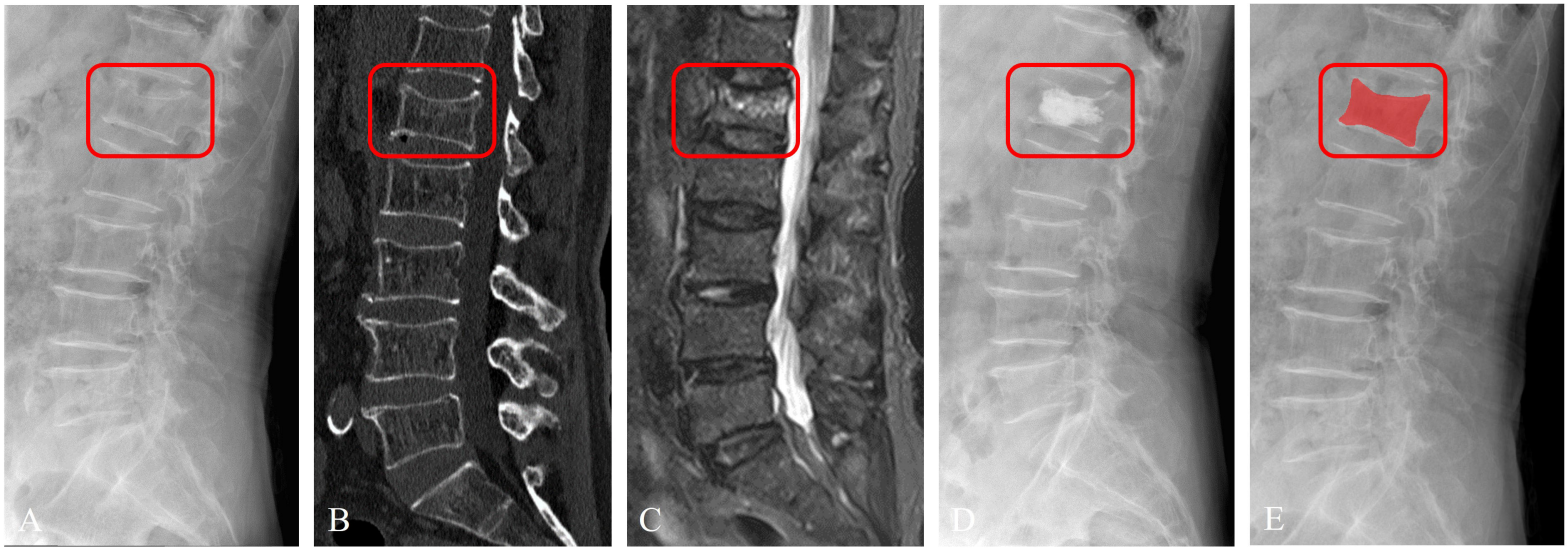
Imaging segmentation of the vertebral body in a 63-year-old female patient diagnosed with acute OVFs, rated an ASTLOF score of 4. The set includes: (A) lateral views on X-ray image, (B) sagittal spine CT image without contrast enhancement, (C) sagittal T2-weighted, fat-suppressed MR image displaying hyperintensity indicative of acute OVFs, (D) lateral X-ray image post percutaneous vertebral augmentation, and (E) ROI identified on lateral X-ray image.
